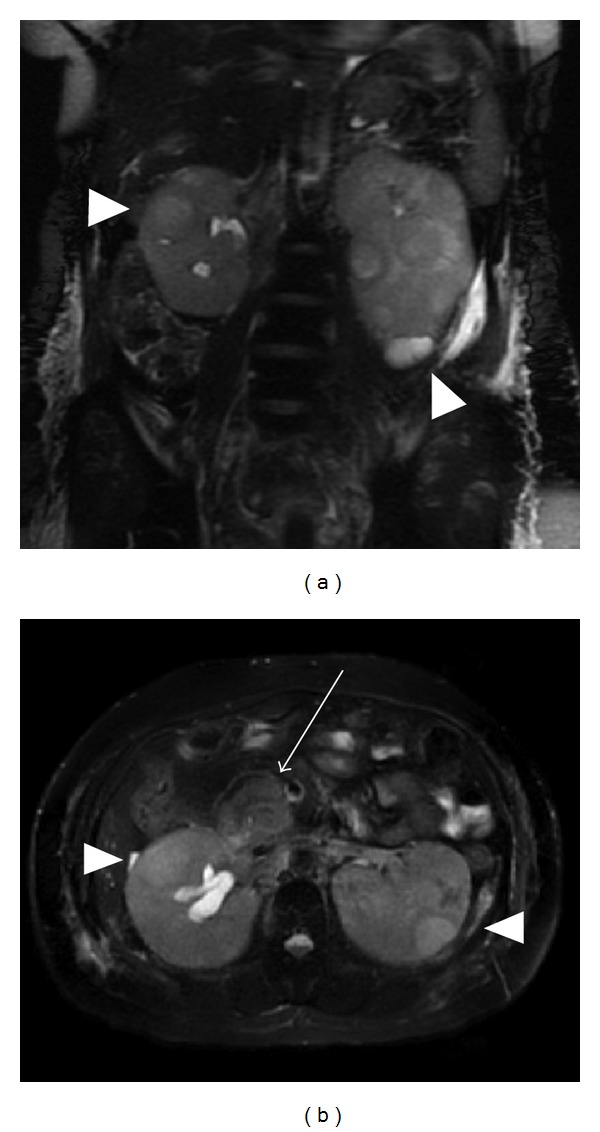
Magnetic resonance imaging of the abdomen showing bilateral renal infiltration. T2-weighted fat-saturated magnetic resonance imaging of the abdomen. (a) Coronal view shows bilateral renal infiltration (arrowheads). (b) Transverse cross-section shows bilateral renal infiltration (arrowheads), unilateral right hydronephrosis, and pancreatic involvement (arrow).
